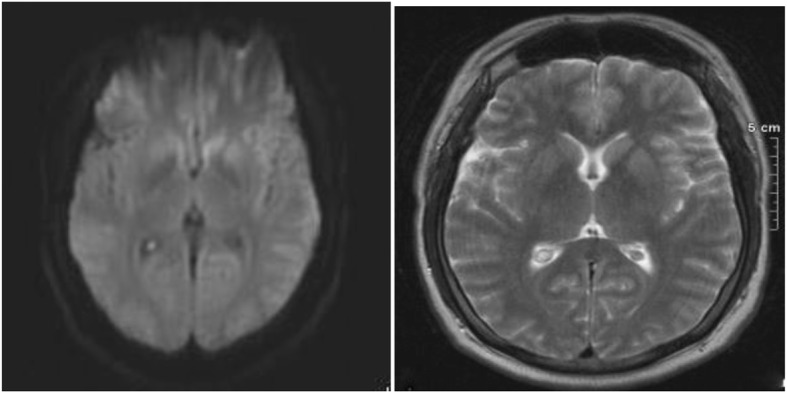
Brain MRI revealed increased DWI and T2 signal in the caudate nuclei (left>right)
